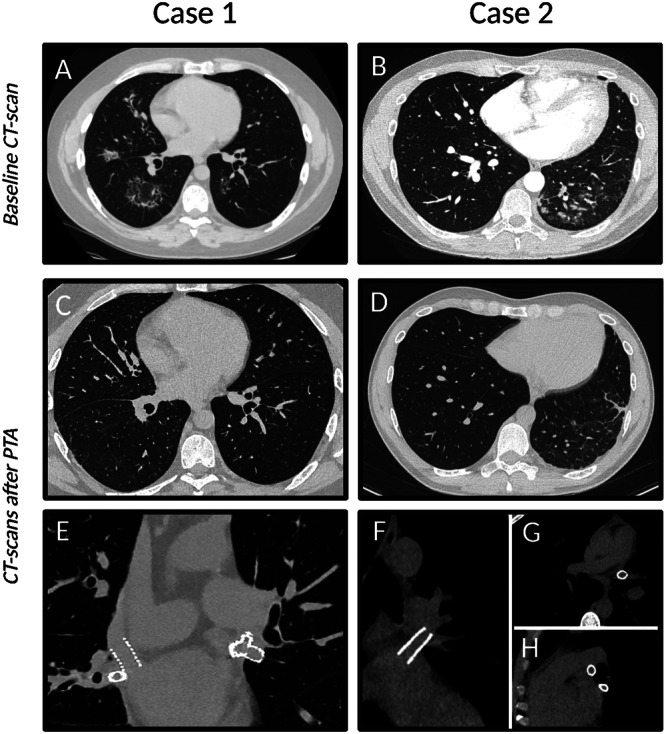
Role of CT in the diagnostic work‐up and follow‐up. In (A), the CT of Patient 1 shows a ground‐glass pattern throughout all the right lobes and involving the left inferior lobe. Also, Patient 2 repeated several CT scans, which constantly showed, as the main sign, a worsening obstructive pneumonia pattern in the left inferior lobe (B). At CT scan follow‐ups, we notice the complete resolution of the obstructive pneumonia pattern for patients 1 (C) and 2 (D). HRCT can offer high‐quality images and be essential to confirm the good results of Pulmonary veins PTA. HRCT of Patient 1 clearly shows the patency of both the stents in RSPV and RMLV, whose origin is separated, and the good result of the culotte technique used to treat the bifurcation of LSPV and LMLV (E). Similarly, we can appreciate the absence of restenosis of the stent in LSPV (F, H) and in the LIPV (G, H) for patient 2.
